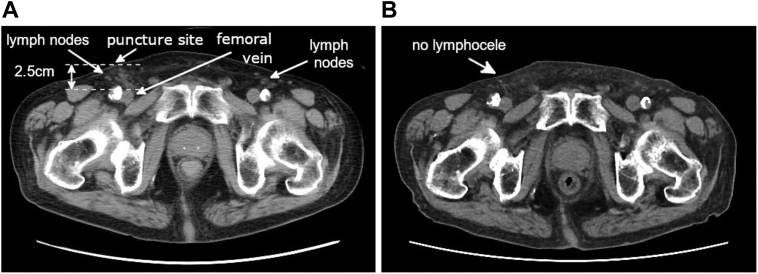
A: CT imaging after the leadless pacemaker implantation revealed multiple lymph nodes in both inguinal regions. However, no lymph nodes suitable for intranodal lymphangiography were identified, and there was no evidence of lymph node injury clearly attributable to the puncture. No anatomic abnormalities were observed in the femoral vessels or lymphatic system. The depth from the skin to the femoral vein at the puncture site was 2.5 cm. B: Follow-up CT imaging at 6 months postoperatively revealed no evidence of lymphorrhea or a lymphocele. CT = computed tomography.
